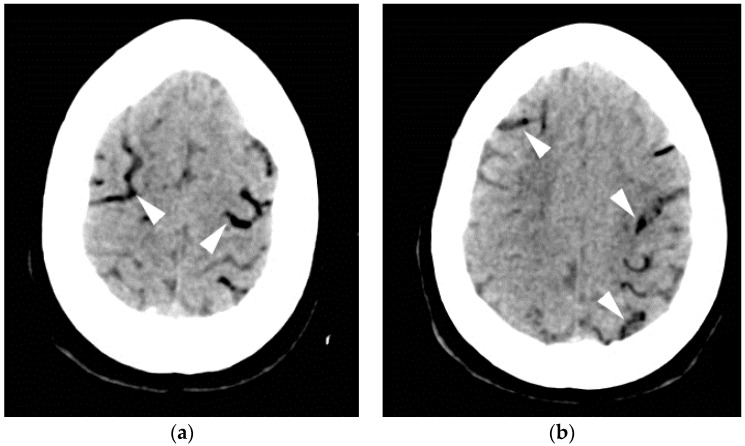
A 27-year-old woman with encephalitis who suffered a respiratory arrest. Two images from axial CT brain (a,b) performed shortly afterward revealed extensive, serpiginous hypodensity in the sulcal distribution overlying the cerebral hemispheres bilaterally (arrowheads), representing intravascular air embolization. The patient made a full recovery with prompt 100% oxygen administration and supportive measures alone.
